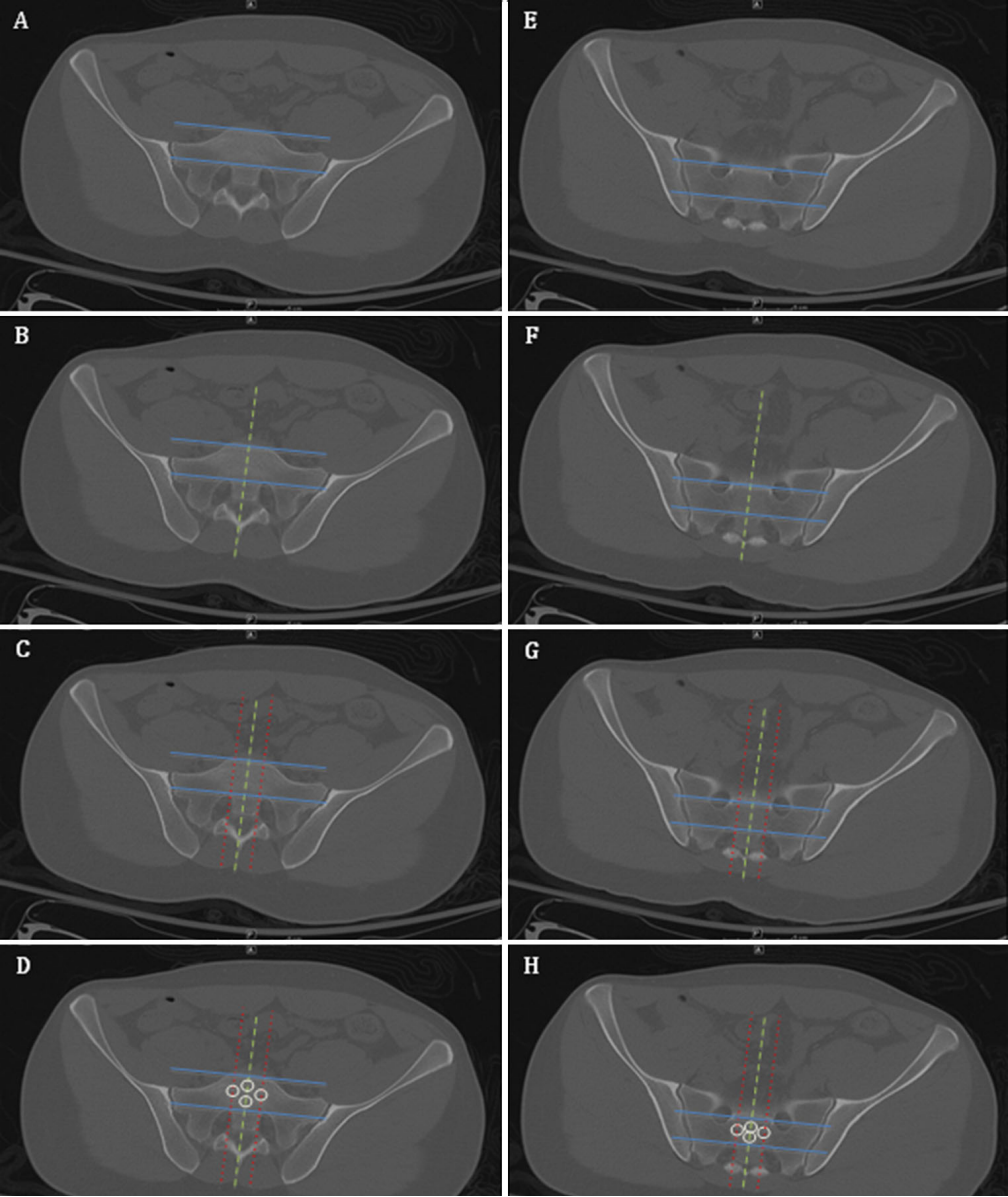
Axial CT sections demonstrating the technique for ROI placement as described in the methods section. a
